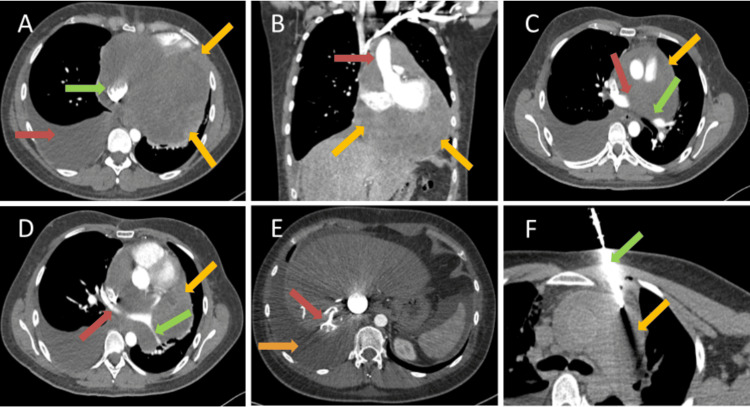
(A) Axial CECT of the lower chest demonstrating a large pericardial mass (yellow arrows in A), encasing the IVC (green arrow in A), with moderate-sized right pleural effusion (red arrow in A). (B) Coronal CECT of the chest demonstrating a large pericardial mass (yellow arrows in B), encasing the ascending aorta (red arrow in B). (C) Axial CECT of the upper chest demonstrating a pericardial mass (yellow arrow in C), encasing the right (red arrow in C) and left (green arrow in C) pulmonary arteries. (D) Axial CECT of the mid chest demonstrating a large pericardial mass (yellow arrow in D), encasing the right inferior (red arrow in D) and left inferior (green arrow in D) pulmonary veins. (E) Axial CECT of the upper abdomen demonstrating reflux of contrast into the hepatic veins (red arrow in E) and right pleural effusion (orange arrow in E). (F) CT-guided biopsy (green arrow in F) of pericardial mass (yellow arrow in F). CECT: contrast-enhanced computed tomography, IVC: inferior vena cava, CT: computed tomography
